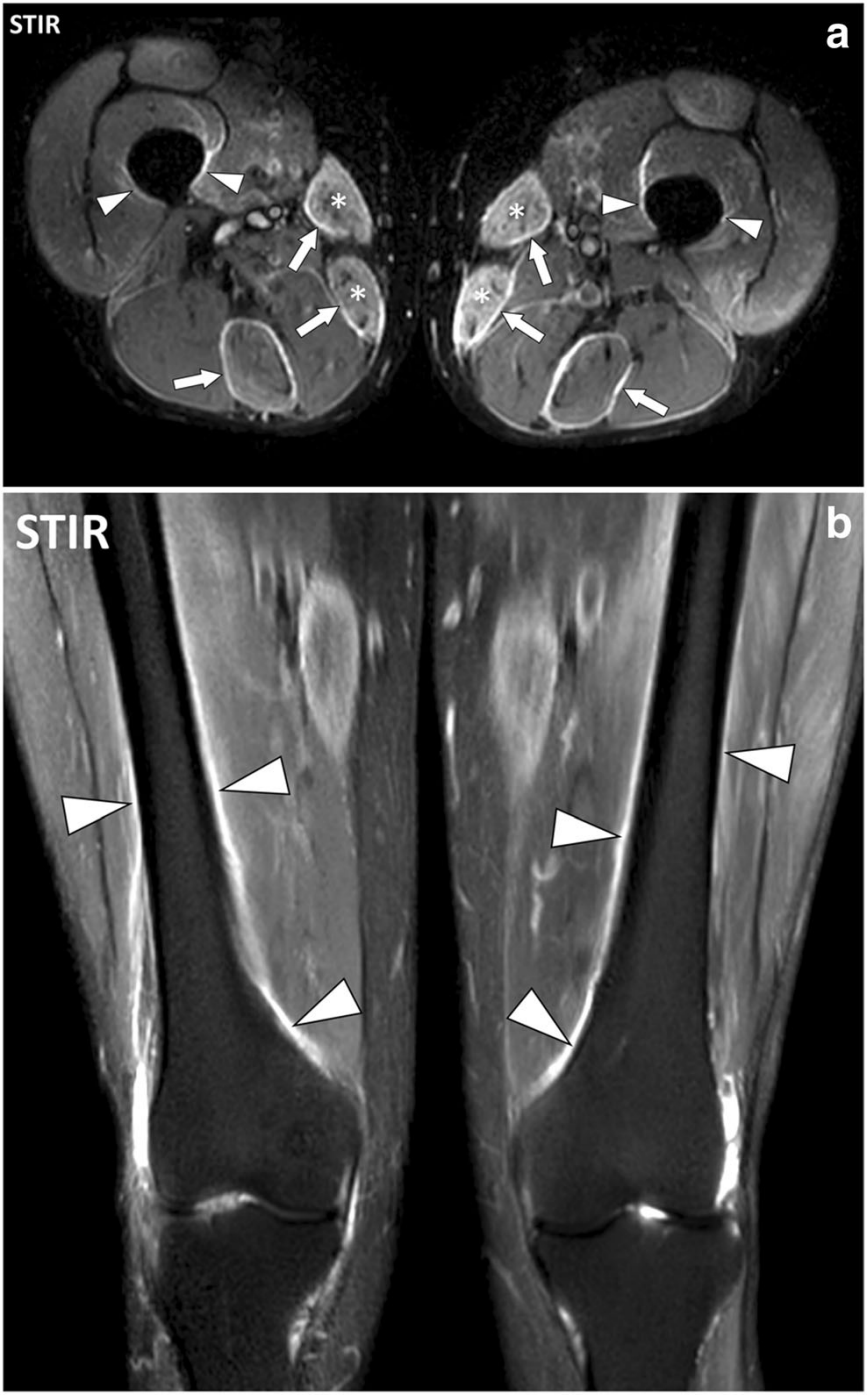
a Axial and (b) coronal STIR images of the thighs of a 51-year-old male with polymyositis. MRI demonstrates bilateral symmetrical involvement with myositis of the sartorius and gracilis muscles (asterisks), fasciitis of the deep fasciae adjacent to sartorius, gracilis and semitendinosus muscles (arrows) and periostitis mostly on the medial part of the diaphysis and metaphysis of the femurs (arrowheads)
